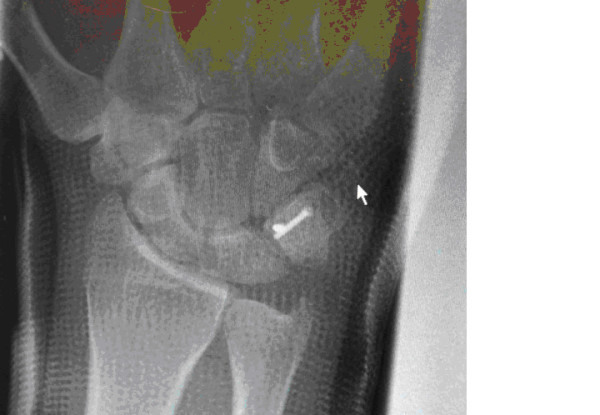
Posteroanterior radiograph of the right wrist at six weeks post-operation. The image shows no evidence of triquetral body non-union, and compression screws *in situ*.
